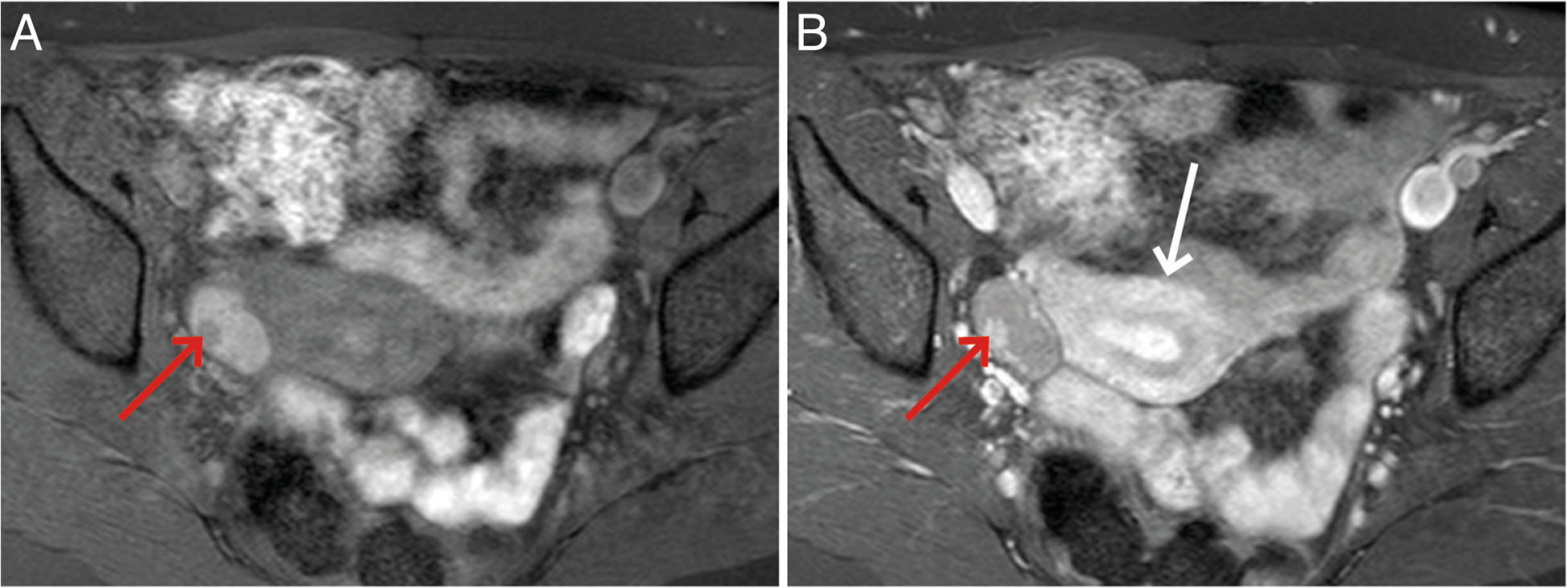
MRI scan of early-stage 1A high-grade serous ovarian cancer. The BRCA1-positive patient was identified through ultrasound screening, confirmed by MRI. The right ovary contains as cyst with an enhancing nodule (red arrows) demonstrated on the T1 fat-saturated image before (a) and after (b) contrast administration. The nodule enhances brightly, similar to the adjacent uterine myometrium (white arrow). Detection of ovarian cancer at this early stage is associated with a significantly improved 5-year survival rate compared with later stages of disease (courtesy: A. Rockall)
